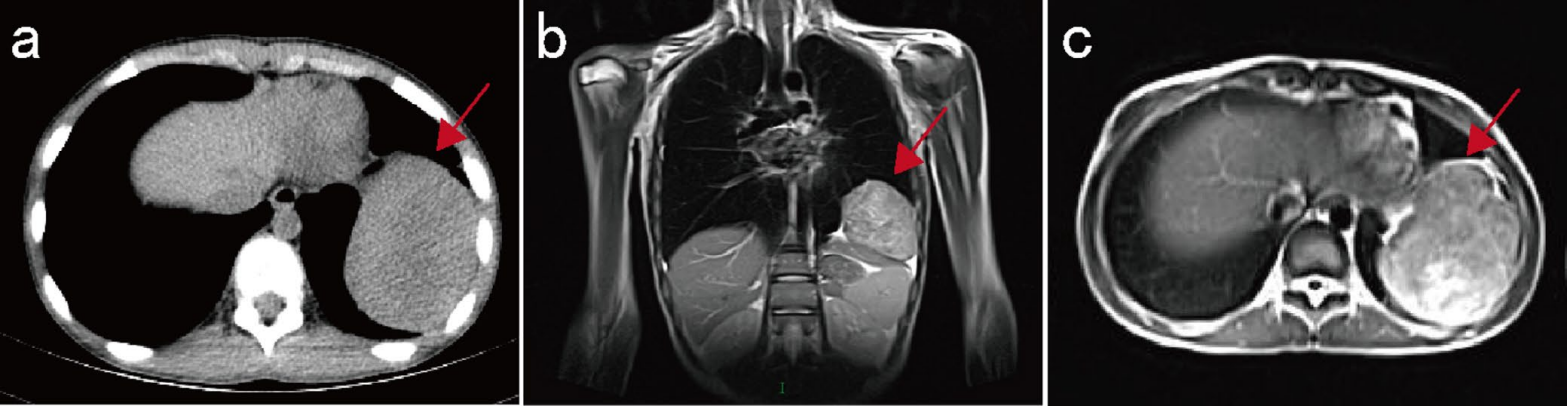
CT and MRI images of the thoracic cavity. (a) Transverse position CT image showing an uneven density and clear border mass located in the left thoracic cavity that is approximately 68 × 88 × 75 mm in size. (b, c) MRI revealed a well‐defined, heterogeneous soft tissue mass shadow located in the left thoracic cavity with localized areas of necrosis and mild compression of the diaphragm. (b) Coronal position, (c) transverse position. Red arrows indicate the mass shadow.
